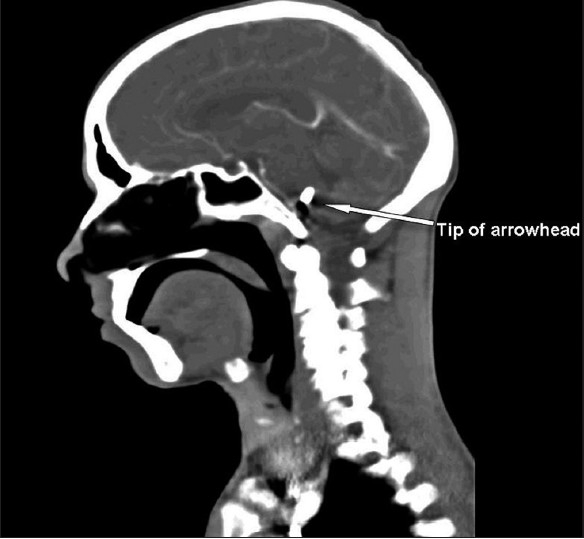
Computed tomography of the brain showing the tip of the arrowhead reaching up to the brainstem
